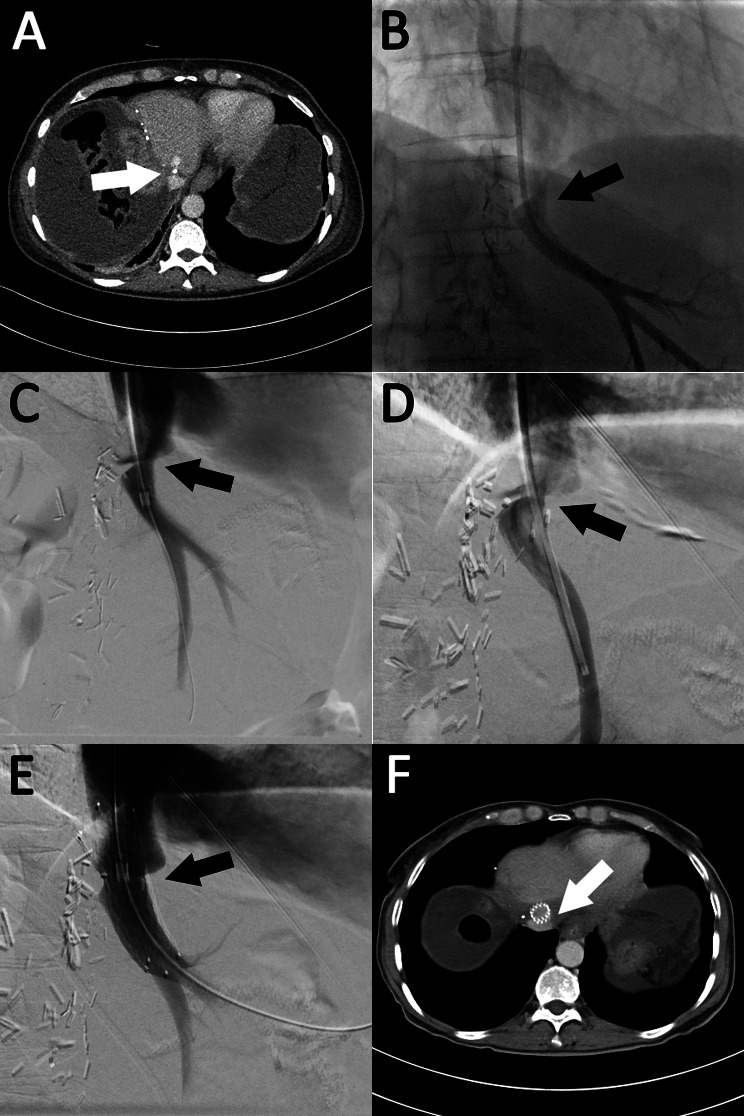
58-year-old male (patient no. 5) presenting with hepatic venous stenosis after extended right hepatectomy for cholangiocarcinoma. A: Axial presurgical CT scan suggesting severe stenotic disease (arrow) at the origin of the left hepatic vein. B and C: Stenosis was found venographically and treated by balloon angioplasty using a 10 mm compliant balloon. D: The stenosis remained present 13 days post first intervention with worsening ascites and therefore it was opted to pursue stent placement utilizing a self-expandable 14 × 40 mm nitinol stent (Sinus repo, Optimed, Ettlingen, Germany) (E). F: CT scan six months post second intervention shows a widely patent stent
